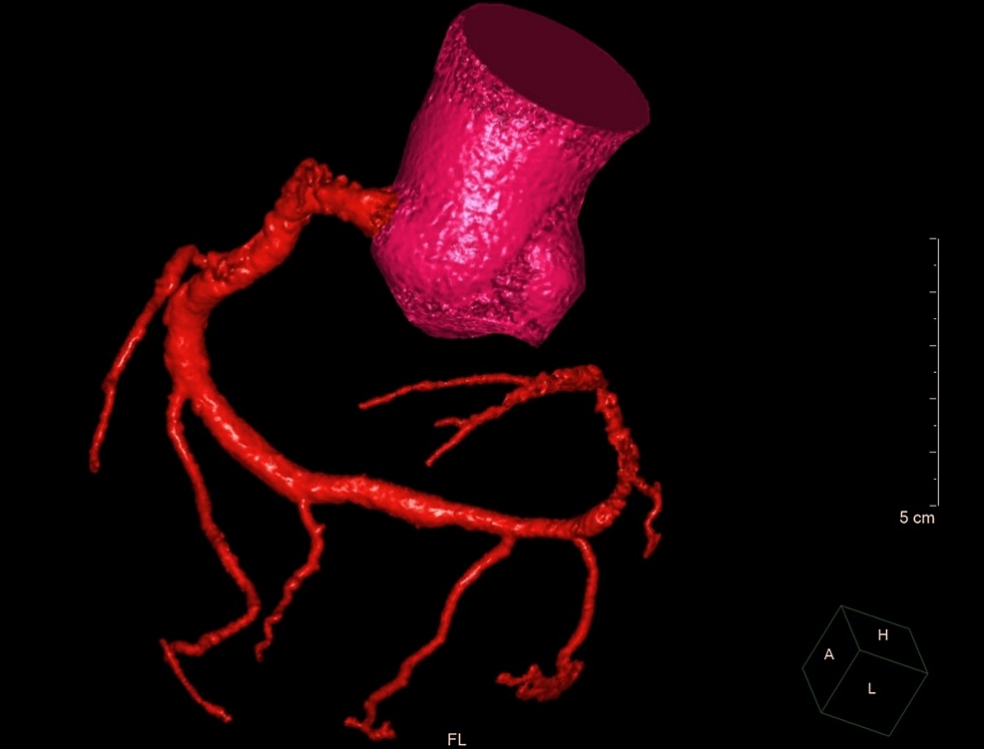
3D CT reconstruction of ascending aorta and single coronary artery CT: computed tomography
